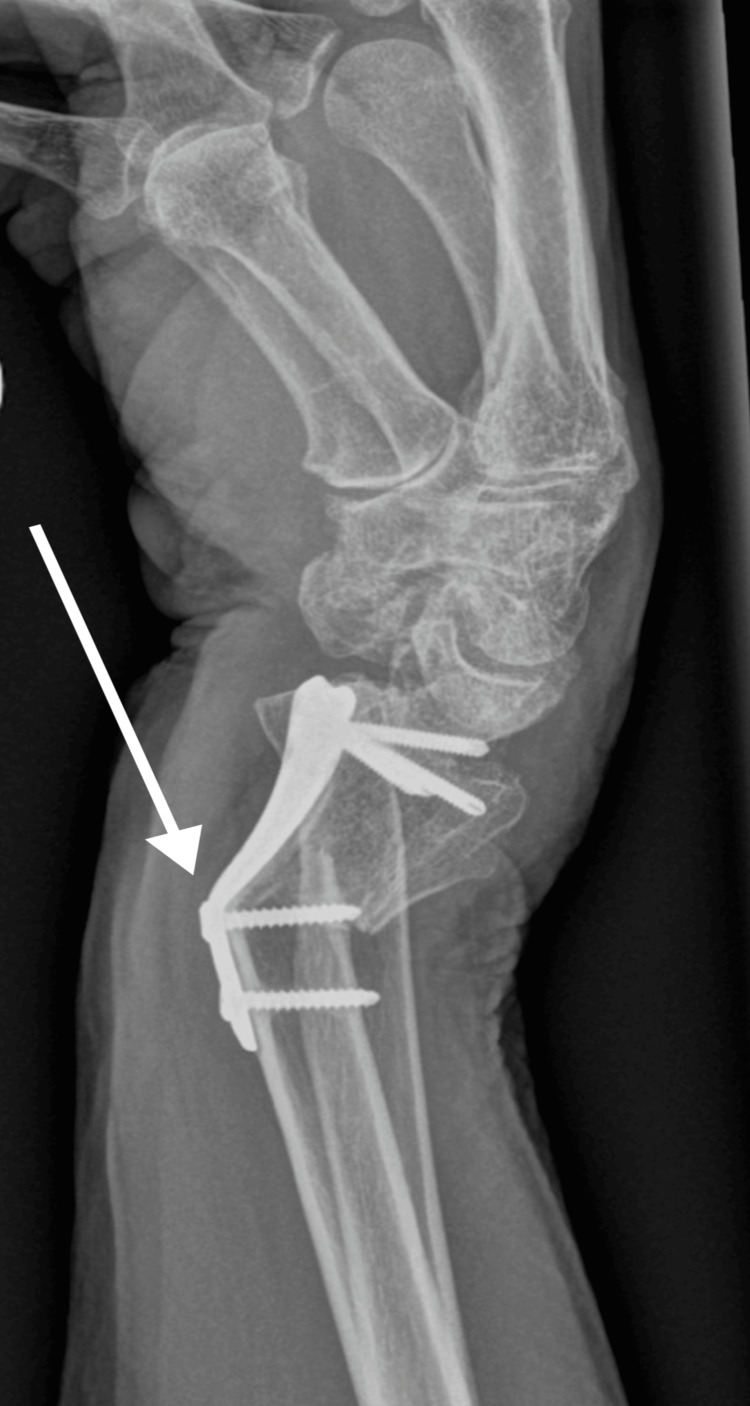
X-ray of radius refracture with bending plate, lateral view
